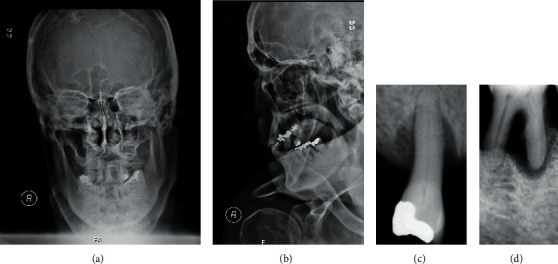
Face X-ray and intraoral X-ray.
